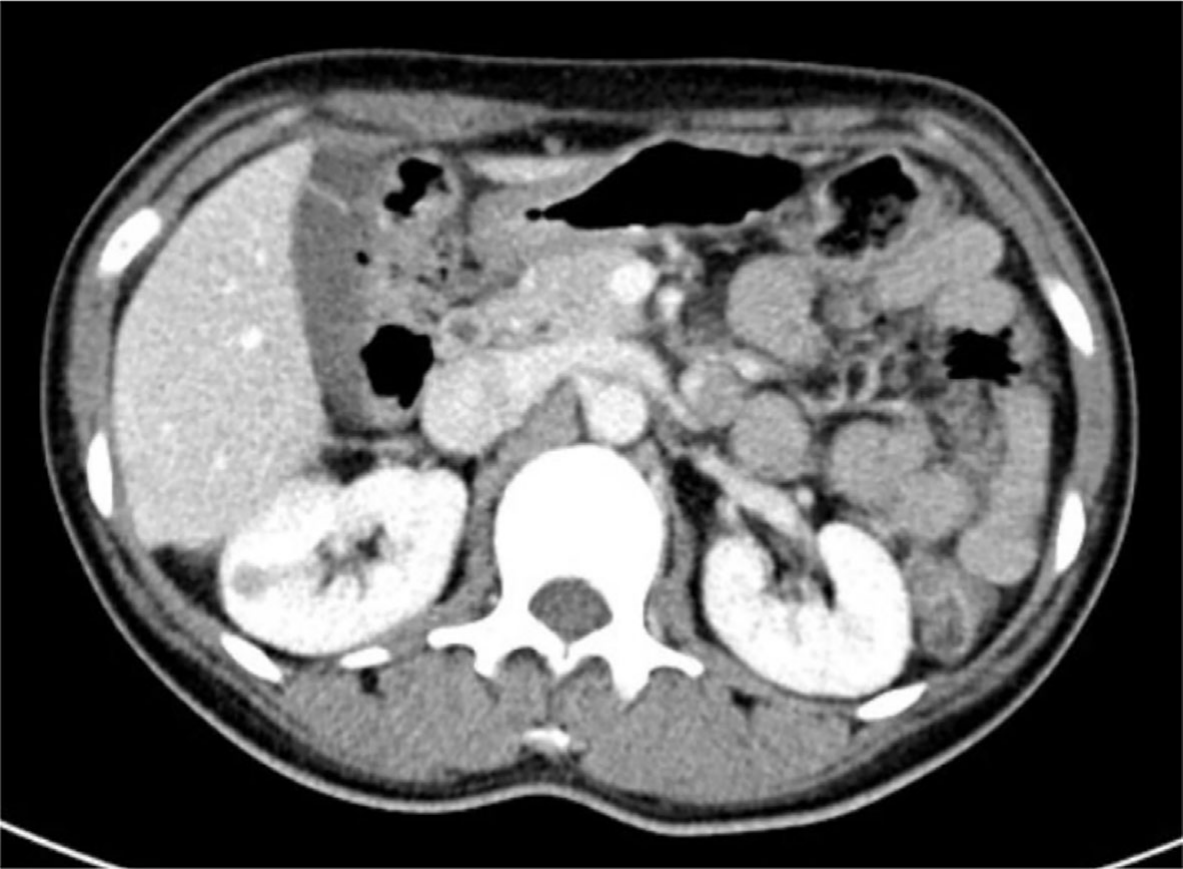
The parenchymal phase of contrast computed tomography image of Patient No. 8.
